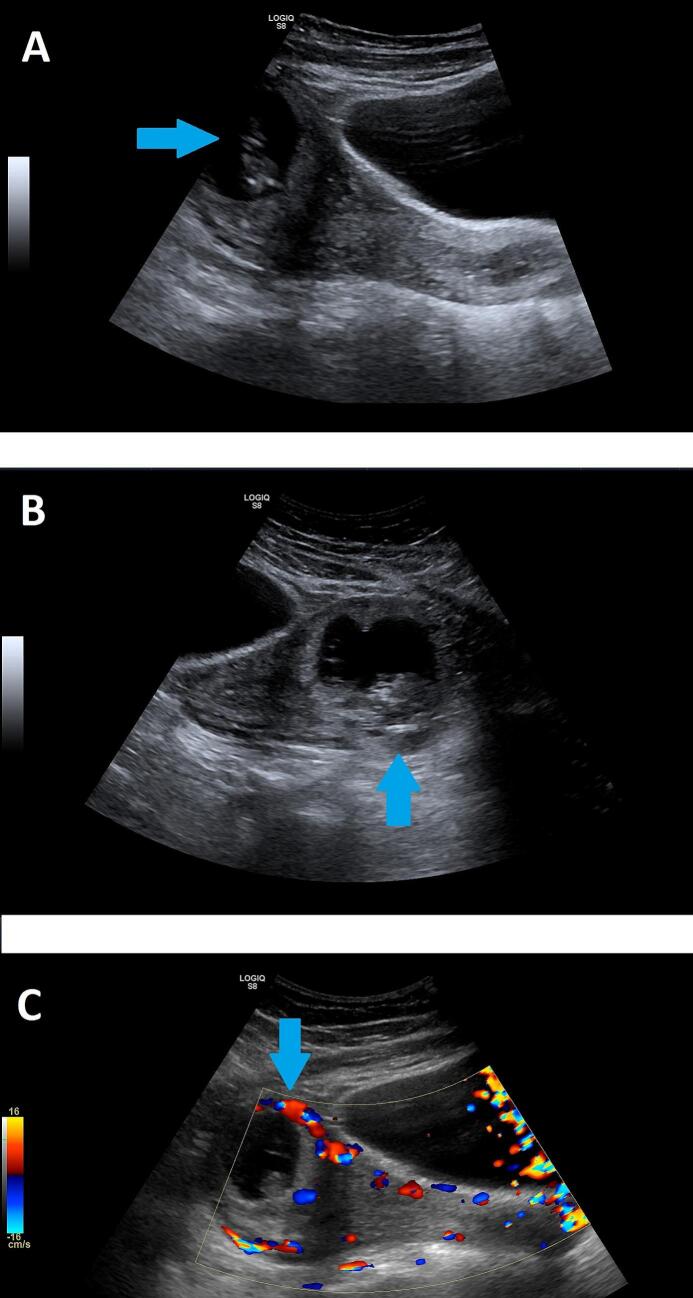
Transvaginal ultrasound of an interstitial ectopic pregnancy. A – Showing a gestation (Blue arrow) near the horn of the uterus. B – Showing a gestation (blue arrow) towards the left side with an empty endometrial cavity. C – Increased vascular Doppler flow (blue arrow) around the gestation.
